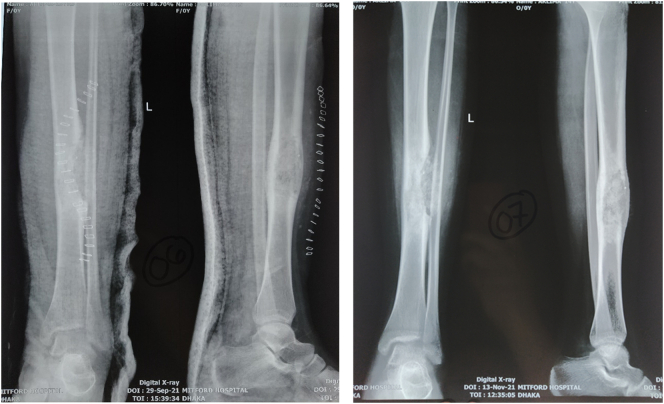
Follow up X-ray on 2nd post-operative day and one and half month after surgery.
